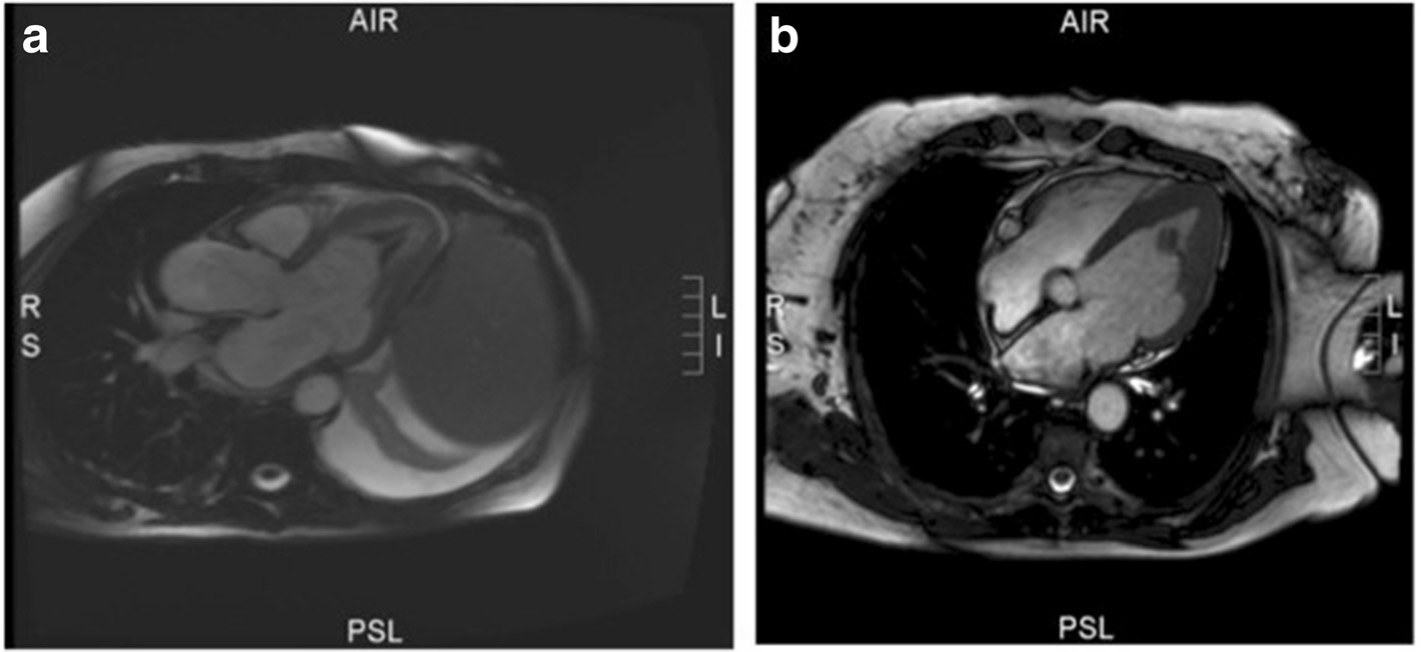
Cardiac MRI (T2-weighted). a: Cardiac MRI showing the thrombus filling the apex. b: Cardiac MRI showing the shrunken, free-floating, and pedunculated thrombus, arising from the lateral wall.
